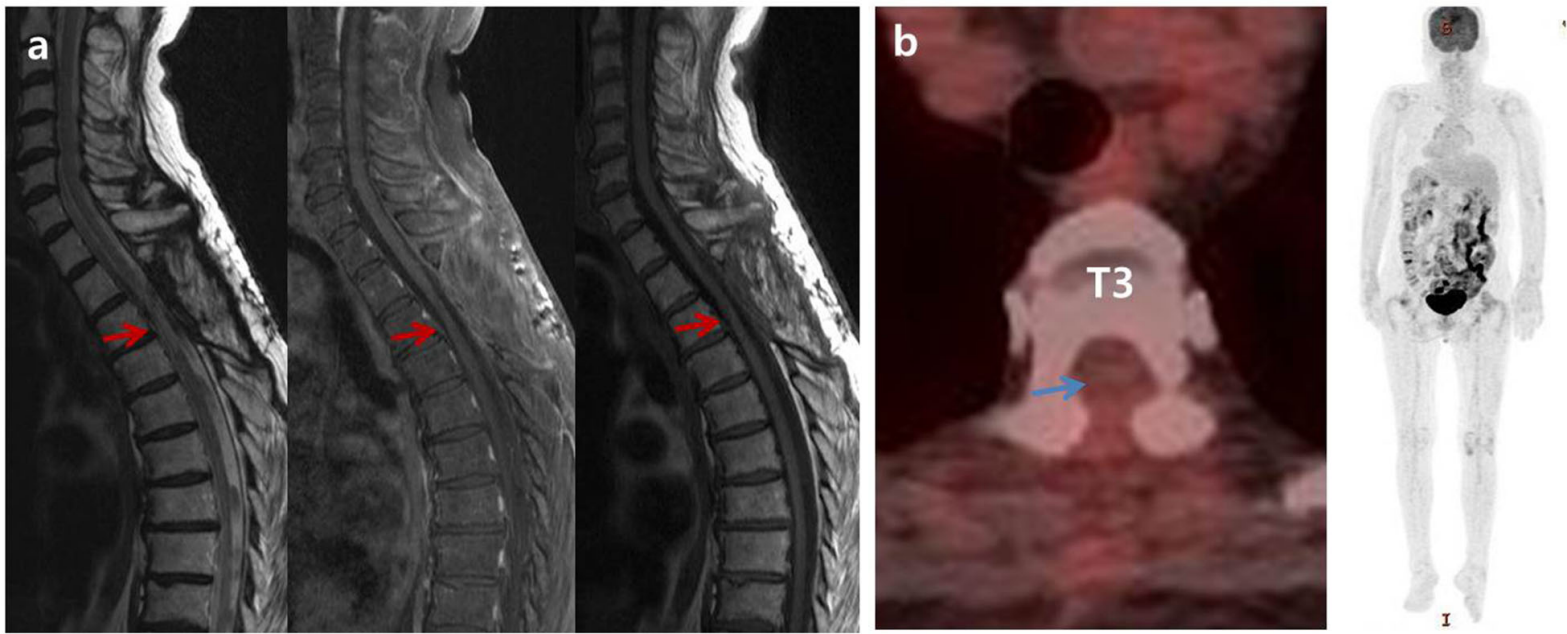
There was no new lesions or recurrence (red and blue arrows) on magnetic resonance imaging (a) and whole-body ^18^F-fluorodeoxyglucose positron emission tomography/computed tomography (b) at 1-year follow-up
